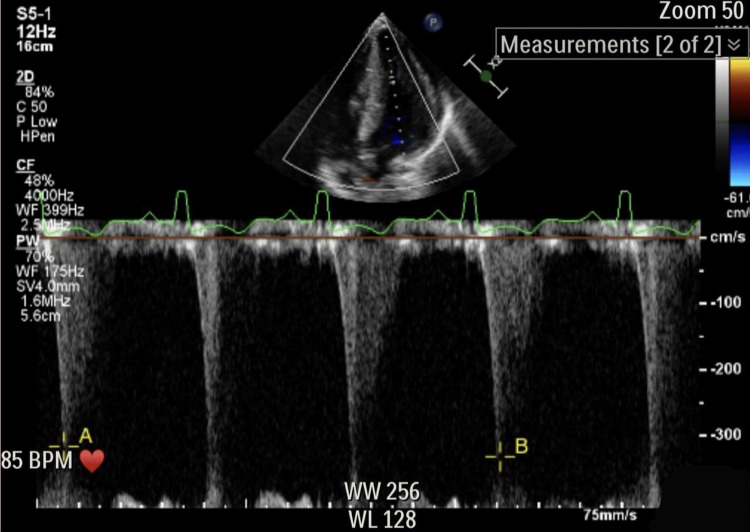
A left ventricle mid-cavity gradient of 36 mmHg through an echocardiogram (ECHO) using a pulse wave Doppler
